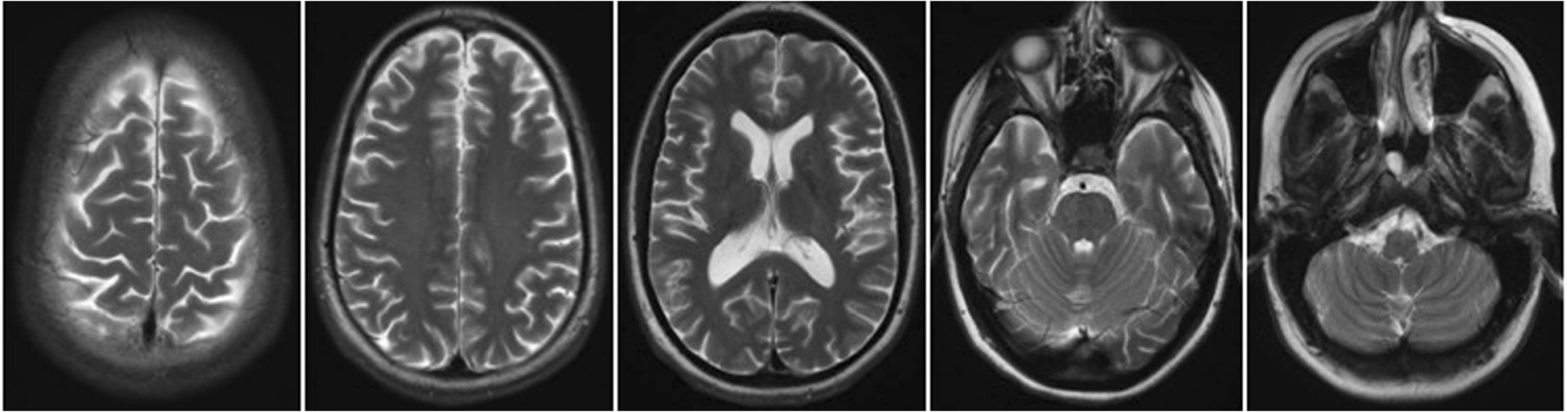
Magnet resonance imaging (MRI) findings. MRI only shows a moderate perisylvic/temporal accentuated atrophy
